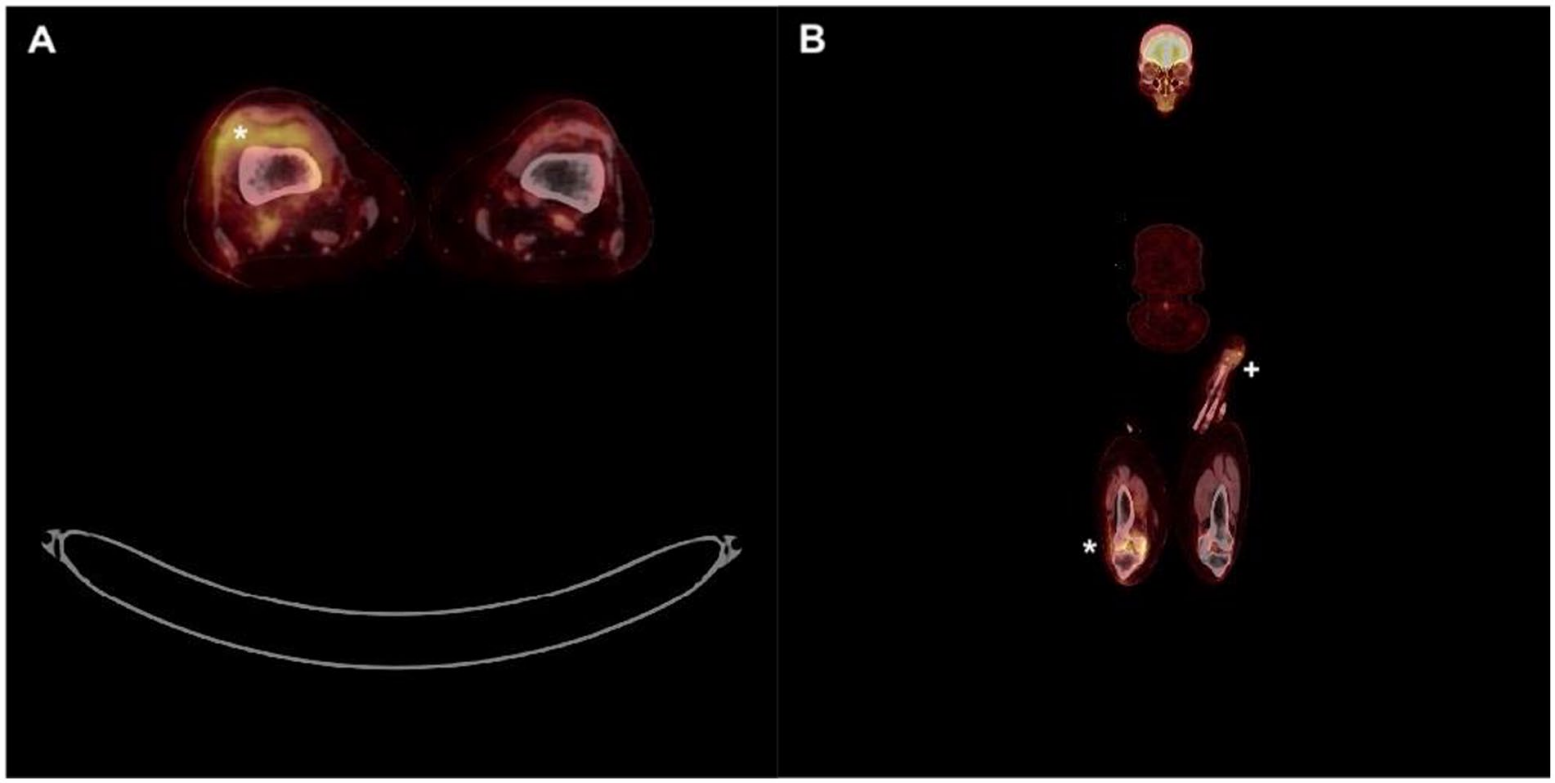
Full body 18F-FDG PET-CT. (A) Hypermetabolic synovitis of the right knee (*). (B) Focal hypermetabolic activity in the left forearm fistula area (+) as well as right knee (*).
